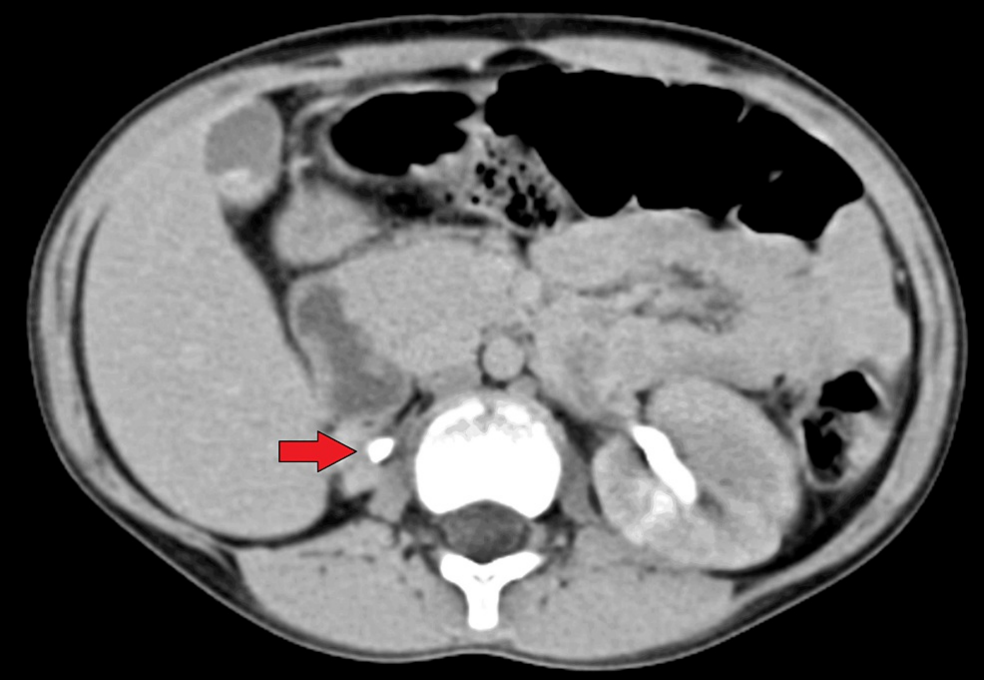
CT urography showing atrophic right kidney. Gross ureter dilatation throughout the course with proximal ureteric diameter 1.6 cm, mid-ureteric diameter 1.7 cm, and distal ureteric diameter 2.4 cm, suggestive of hydroureter
